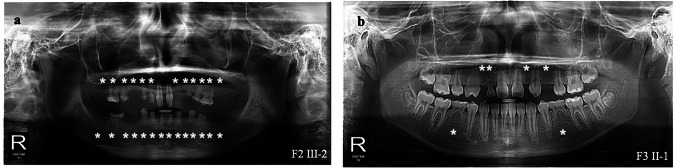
Panoramic radiographs of the two participants in the Family 2 and Family 3. a F2 III-2 15 years old girl is missing 26 permanent teeth, conically shaped maxillary incisors, and primary teeth still remain. b F3 II-1 15 years old girl is missing 6 permanent teeth and 52, 75, and 85 still remained
